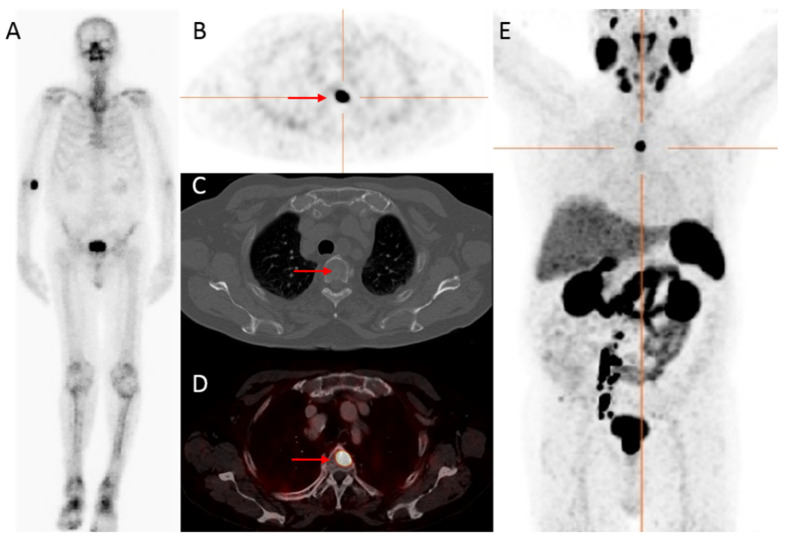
An 83-year-old patient, with castration-resistant prostate cancer (CRPC) after initial treatment with hormonal therapy (2009), and secondary abiraterone (2019), showed improved detection of bone metastatic prostate cancer PCa with ^68^Ga-PSMA PET/CT compared to bone scintigraphy. The prostate—specific antigen (PSA)—value at PET scanning was 25.9 ng/mL. On the bone scintigraphy, no suspect bone metastases were visualized (A). Transversal ^68^Ga-PSMA PET (B), fused PET/CT (D) and maximum intensity projection (MIP) (E) revealed a lesion located in the thoracic spine with increased PSMA expression (red arrow), with no evident substrate on CT (C). Time interval between bone scintigraphy and ^68^Ga-PSMA PET/CT was 5 weeks.
